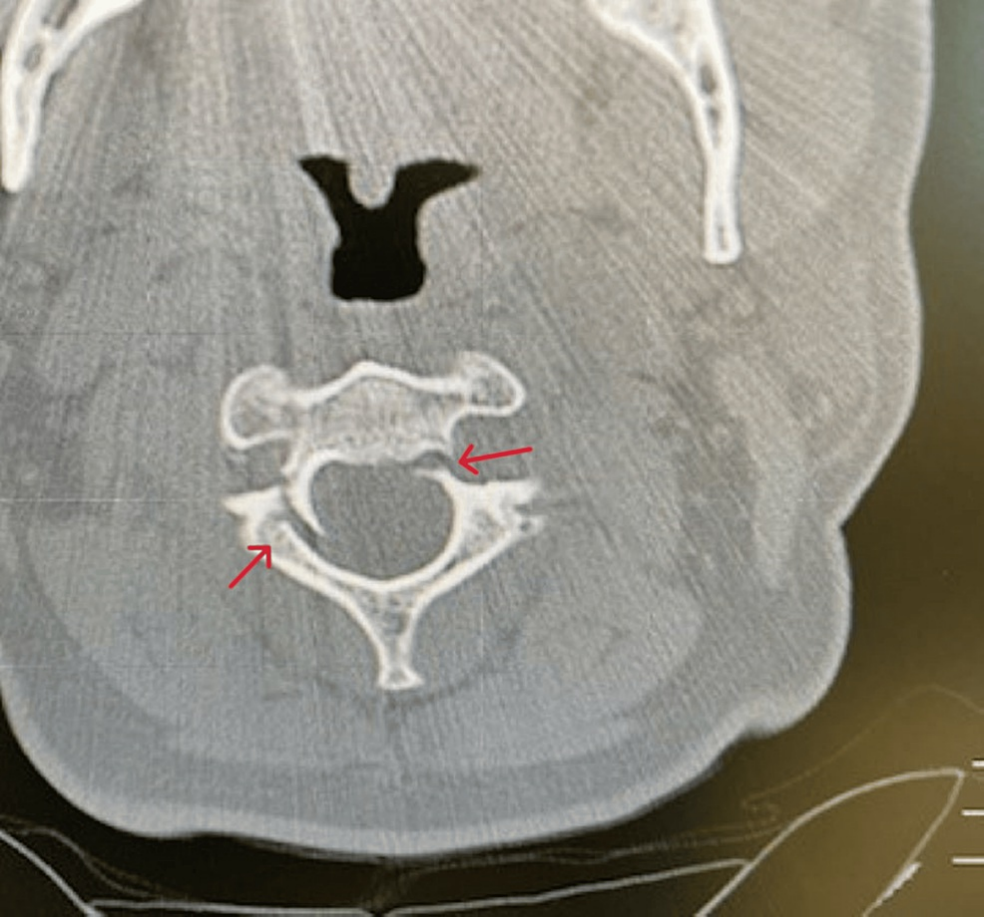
Non-contrast CT (transverse view) of the C2. Note the bilateral fracture separating the lamina and body.
